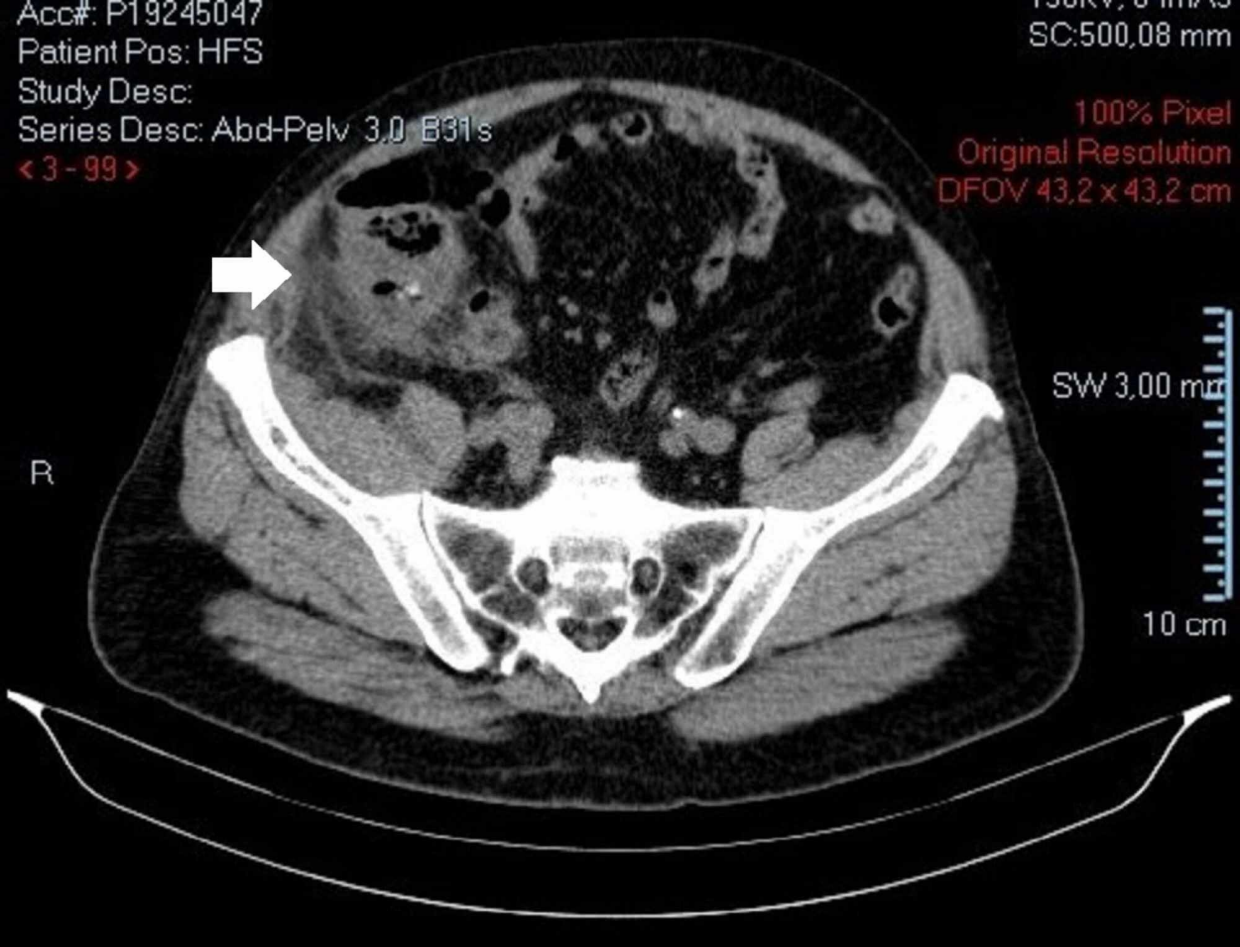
Abdominopelvic CT scan. Fat stranding and parietal peritoneal thickening in parietocolic gutter.
